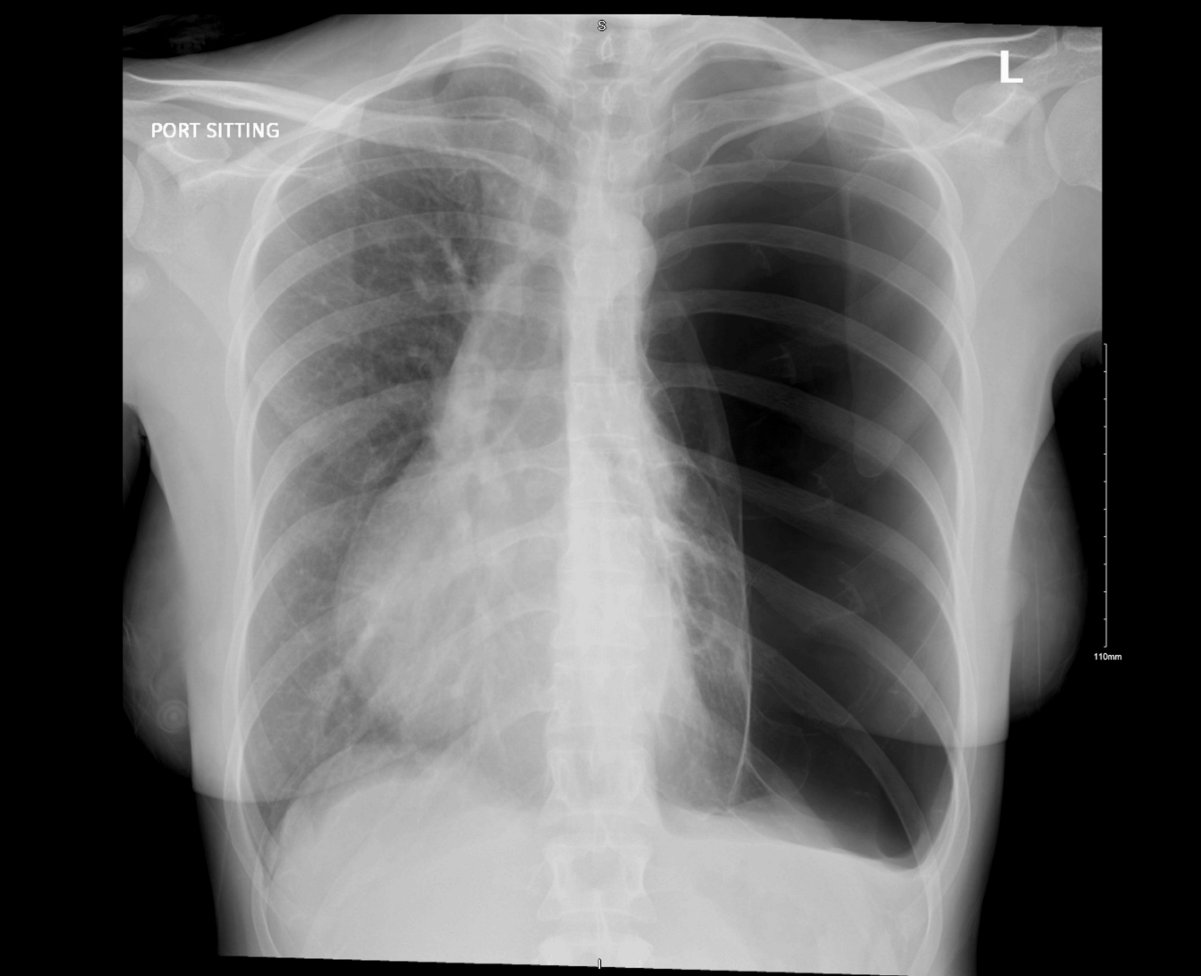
Preoperative chest X-ray showing a large bulla on the left side
